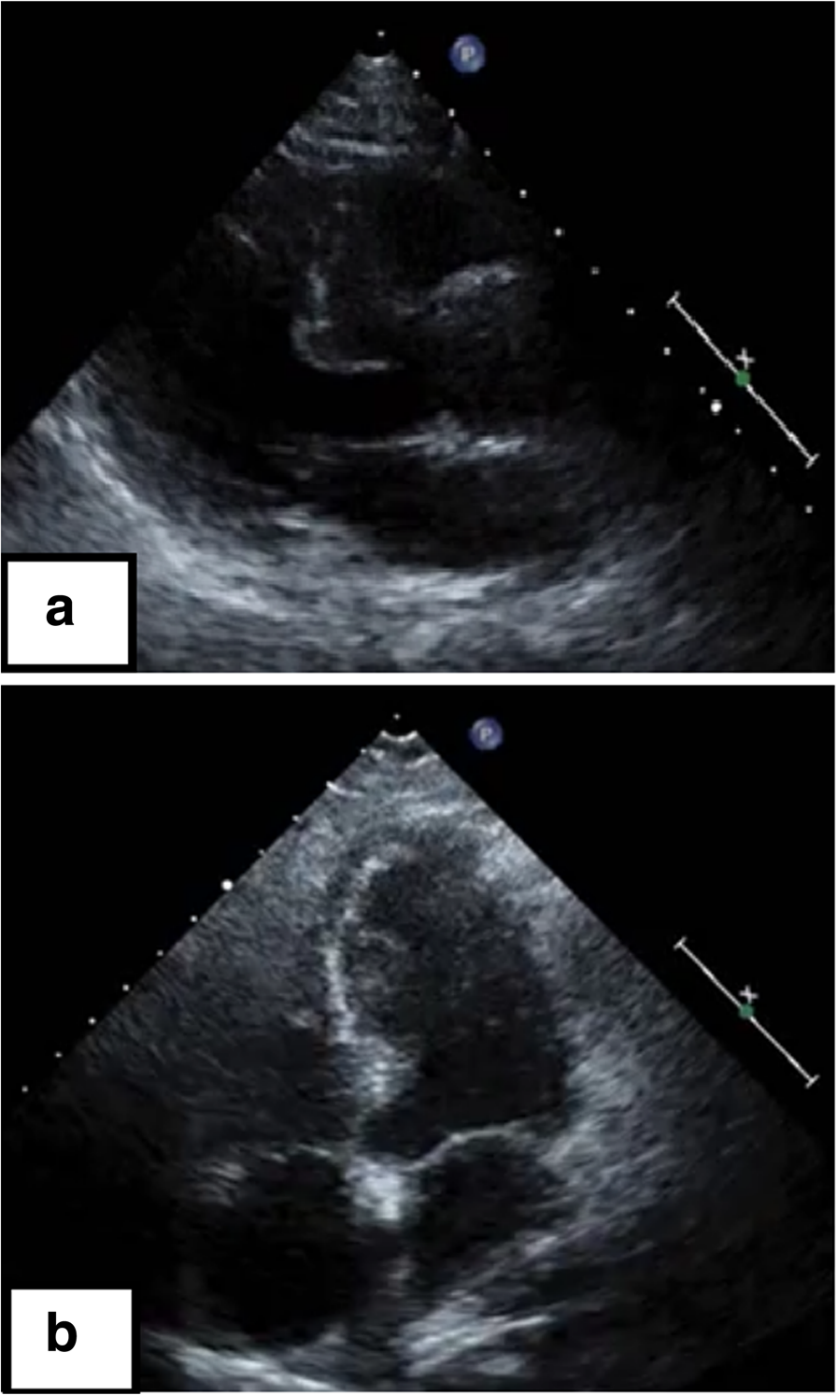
Transthoracic echocardiography on day 10. In the parasternal long axis view (a) and four-chamber view (b), echocardiography revealed a morphological characteristic of the basal interventricular septum that protrudes into the left ventricular cavity
